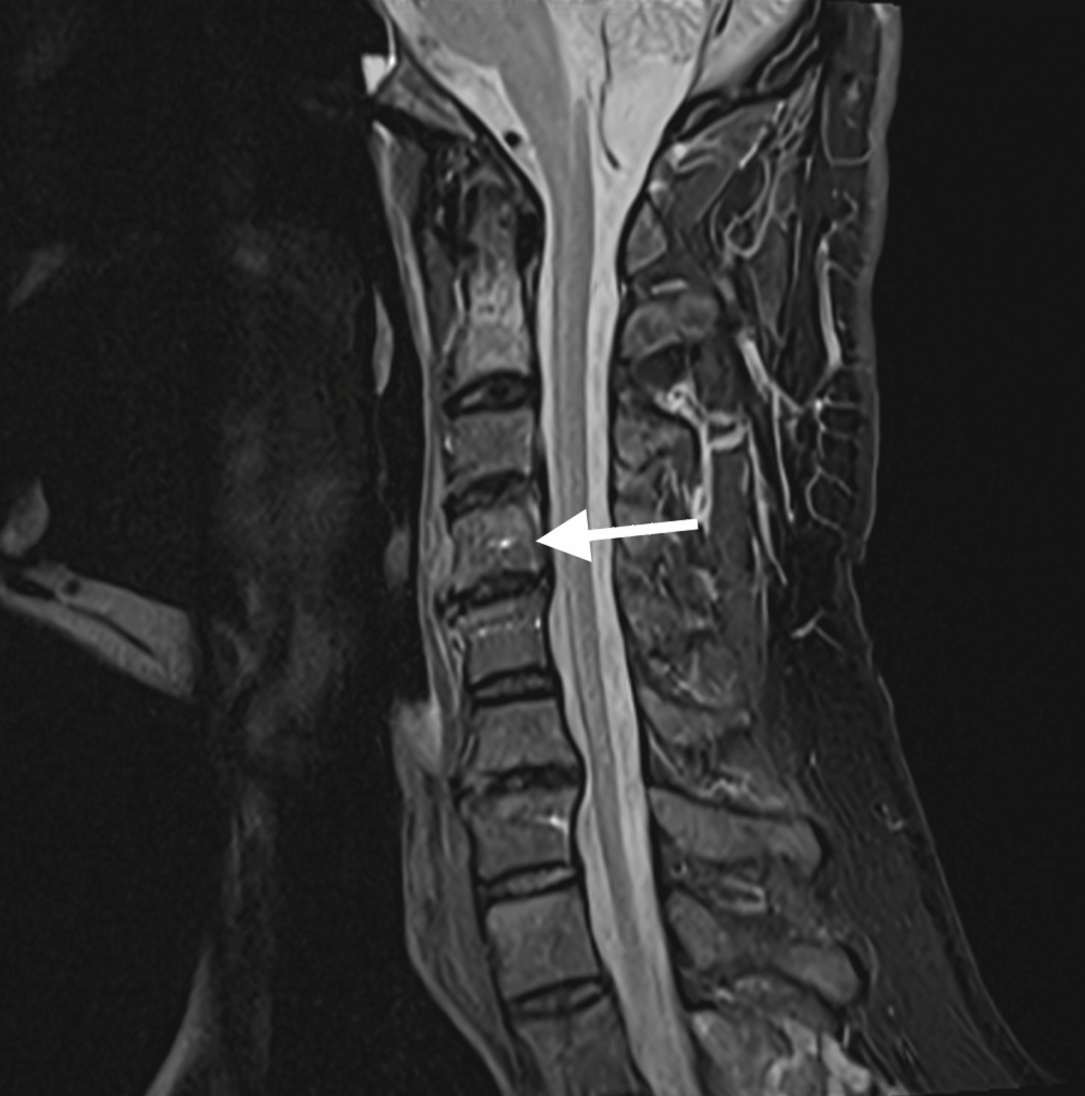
Spine MRI The image demonstrates patchy areas of abnormal marrow signal and contrast enhancement involving the cervical vertebrae, most prominently at the C4 vertebral body (arrow). MRI: magnetic resonance imaging
